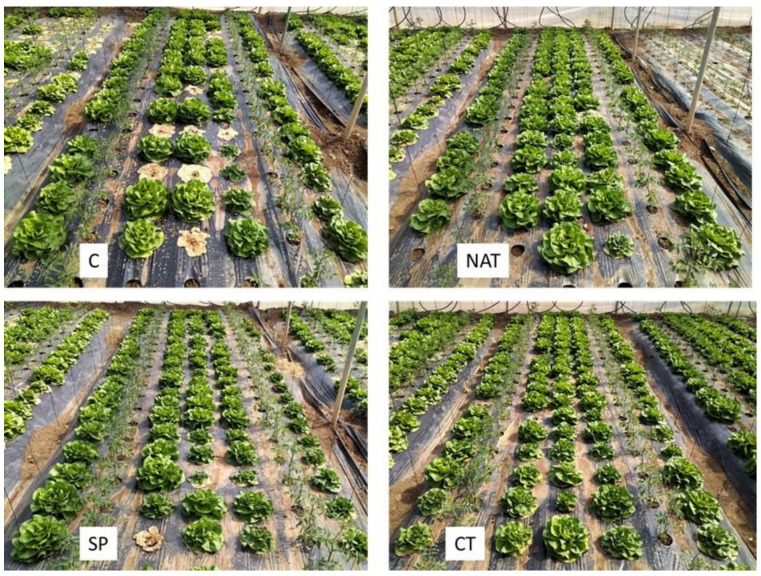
Examples of experimental plots of *Lactuca sativa* organic fertilization tests: Control (C) irrigated only with water and showing higher occurrence of *Fusarium oxysporum* attack. Treatments by foliar application with water with added spirulina (SP), spirulina after natural uptake of *F. oxysporum* DNA (NAT), and commercial compost tea (CT).
